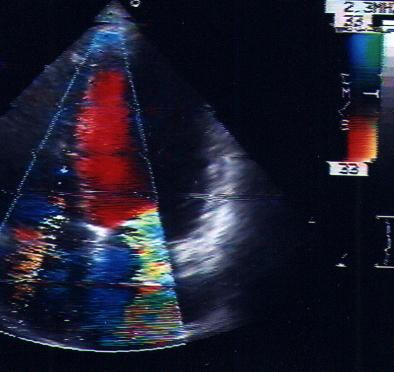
TEE showed, on apical 4-chamber section, an anular structure attached like a membrane to the atrial wall anterior mitral valve leaflet and just proximal to the posterior mitral leaflet.
